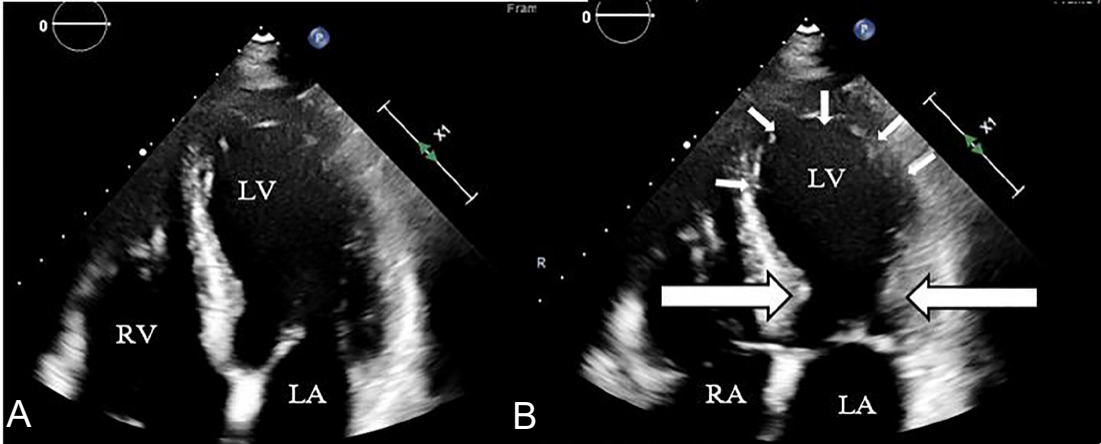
Point-of-care ultrasound images in the apical four-chamber view: A) diastole and B) systole demonstrating normal to hypercontraction of the base of the left ventricle (large arrows) and a hypodynamic apex (small arrows). *LV,* left ventricle; *RV,* right ventricle; *LA,* left atrium; *RA,* right atrium.
